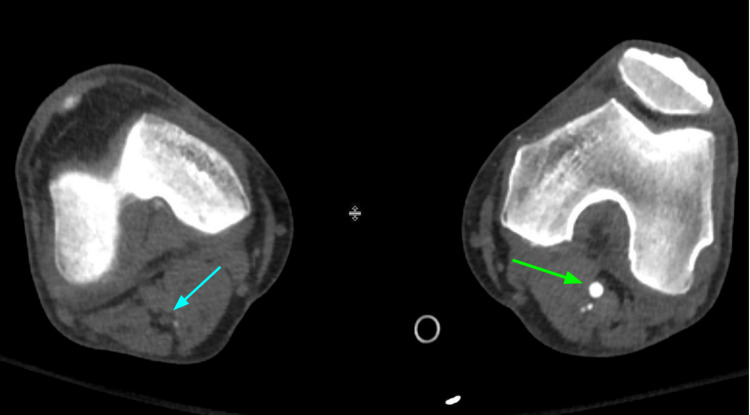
The same patient with popliteal arterial thrombosis on the right. Non-enhancement of the right popliteal artery (blue arrow) when compared to the left in this lower extremity (green arrow) CT angiogram is shown.
